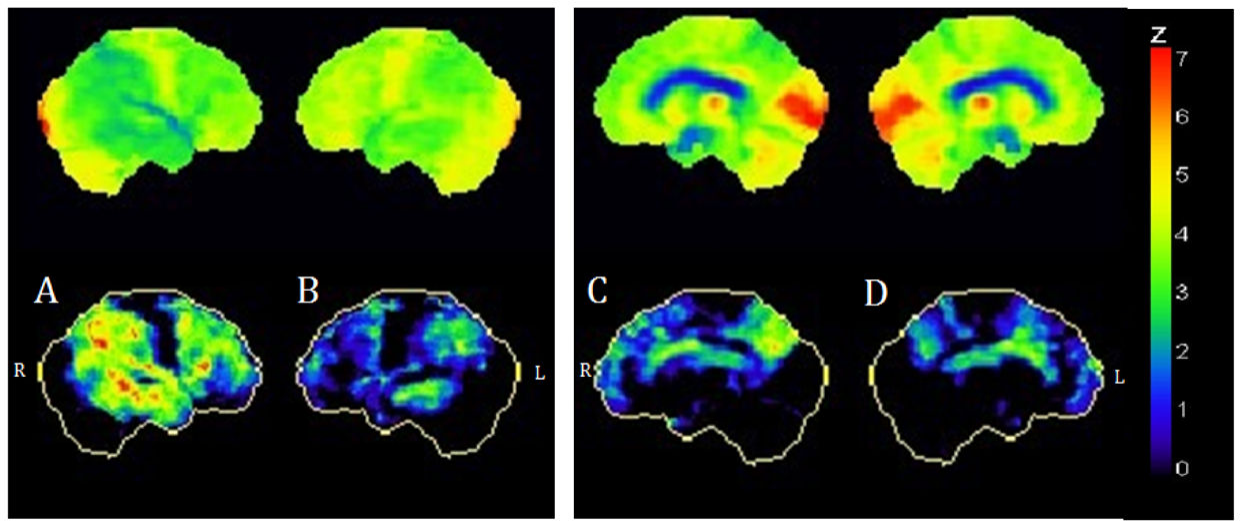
FDG-PET of the brain (A) and (B) show asymmetric bilateral fronto-temporo-parietal hypometabolism, more severe on the right while (C) and (D) show sparing of the posterior cingulate gyrus.
